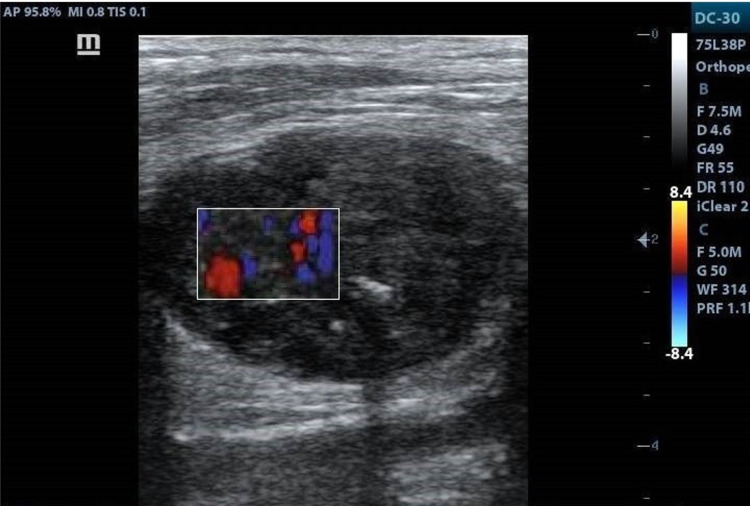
Doppler ultrasonography image demonstrating color uptake within the lobulated hypoechoic lesion consistent with features of central vascularity.
